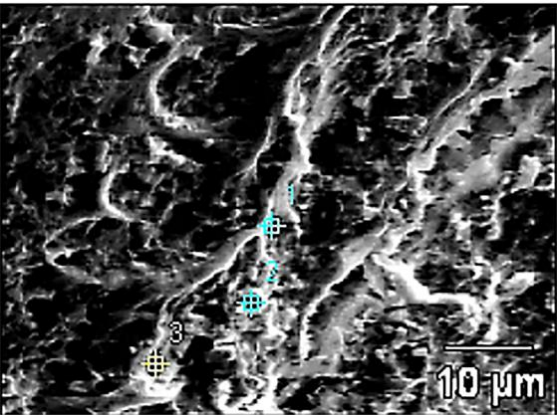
Mineral composition analysis by Energy-Dispersive X-ray
